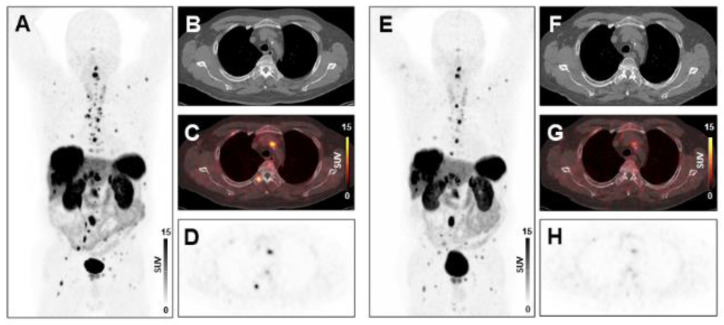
Treatment response to peptide receptor radionuclide therapy (PRRT) in a patient with low systemic inflammation at baseline. Maximum-intensity-projection (MIP) image (A) and transversal PET/CT images (B–D) of baseline [^68^Ga]Ga-DOTA-TATE PET/CT showing osseous, hepatic, and lymph node metastases in a 66-years-old male gastro-entero-pancreatic neuroendocrine tumor (GEP-NET) patient. MIP image (E) and transversal PET/CT images (F–H) at follow-up after two cycles of PRRT demonstrating decreasing tracer uptake or disappearance of lesions (SSR-TV decreased from 496 cm^3^ to 277 cm^3^, consistent with stable disease). The baseline CRP level was 1.6 mg/L, ANC was 4.0 × 10^9^/L, the PCM was 227.2, and the HSPI was 0, evidencing low systemic inflammation.
